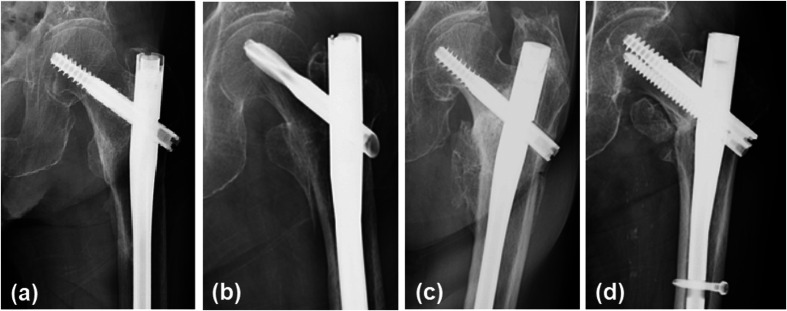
These radiographs show the deformity degree. (a) The radiograph presented mild deformity but showed complete bone union. Slight ossification was observed around the tape. (b) The radiograph presented moderate deformity but also showed complete bone union with 5–10 mm displacement of the lesser trochanter fragment. (c) The radiograph presented severe deformity with the displacement of lesser trochanter fragment to >1 cm from the base. (d) The radiograph showed ununited fragment. The displaced fragment shifted from the original position and was observed in the anterior proximal aspect of the femur. This may be affected by the iliopsoas muscle force.
